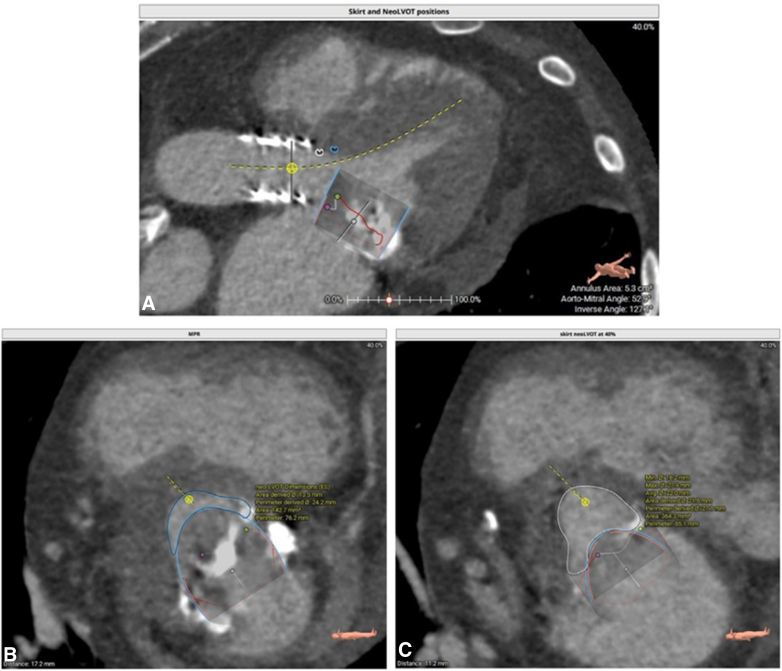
LVOT obstruction after transcatheter mitral valve replacement is a possible complication of transcatheter mitral valve replacement. A, CT reconstruction of LVOT in a patient with a THV with virtual implantation of SAPIEN valve in the mitral position. B, Assessment of neo-LVOT. C, Skirt-to-neoLVOT in 40% phase. *CT*, Computed tomography; *LVOT*, left ventricular outflow tract; *THV*, transcatheter heart valve.
